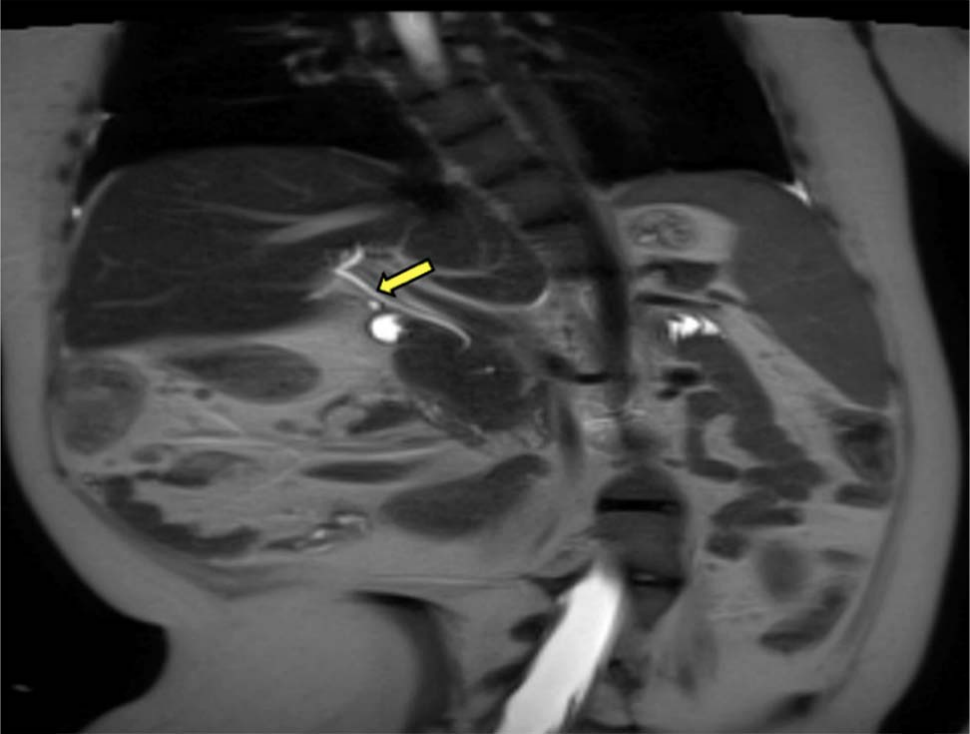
HASTE MRI showing a smooth, nondilated common bile duct (yellow arrow). HASTE, half Fourier single-shot turbo spin-echo; MRI, magnetic resonance image.
